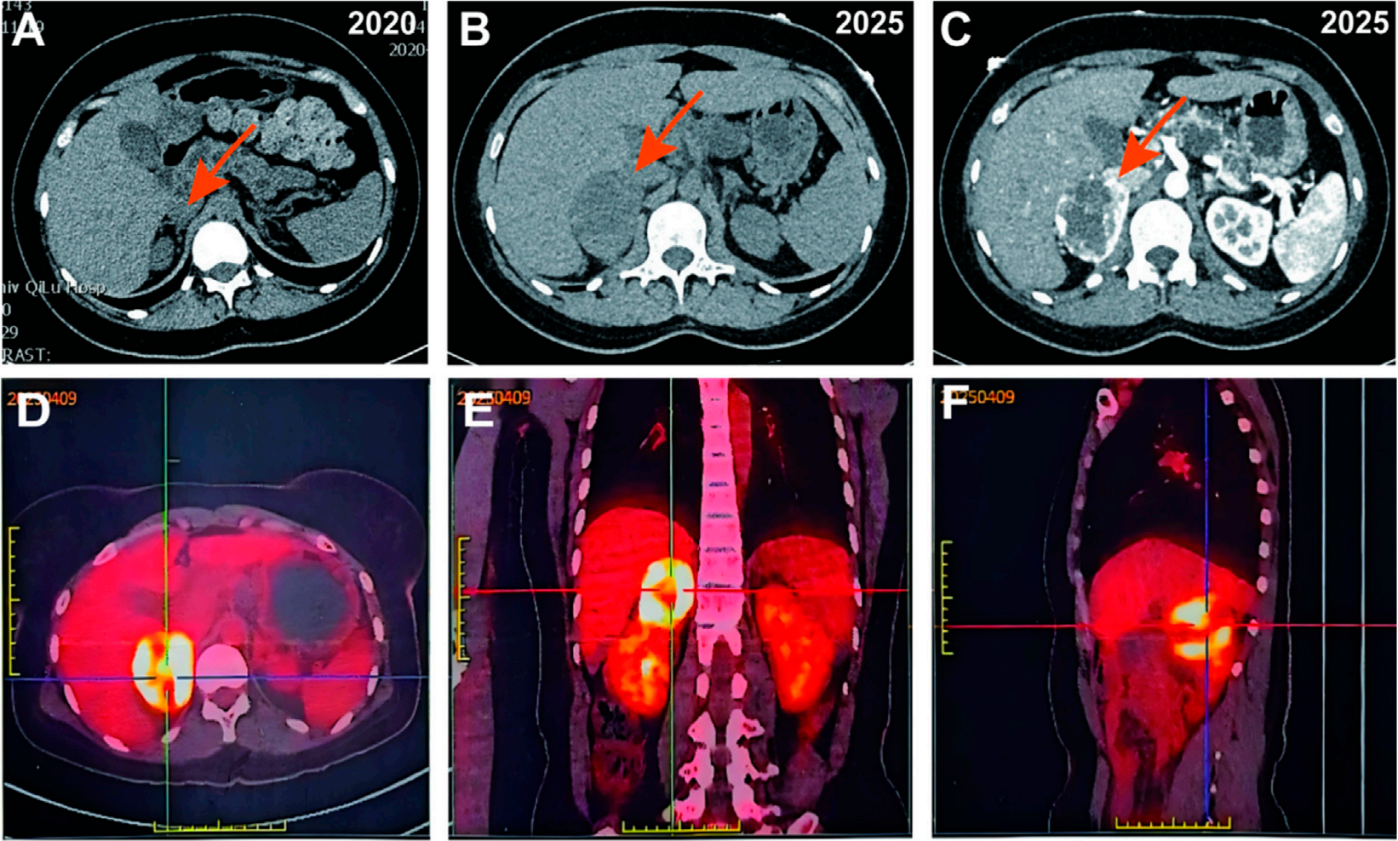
Adrenal gland imaging showing a space-occupying lesion in the left adrenal gland. (A) Adrenal glands in 2020. (B,C) Tumor of the right adrenal gland in 2025. (D–F) High-intensity FDG uptake of the mass in the right adrenal area in PET-CT.
